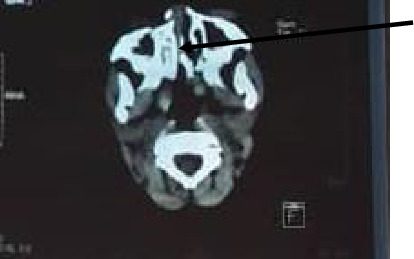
Axial view of CT scan showing sagittal fracture.
